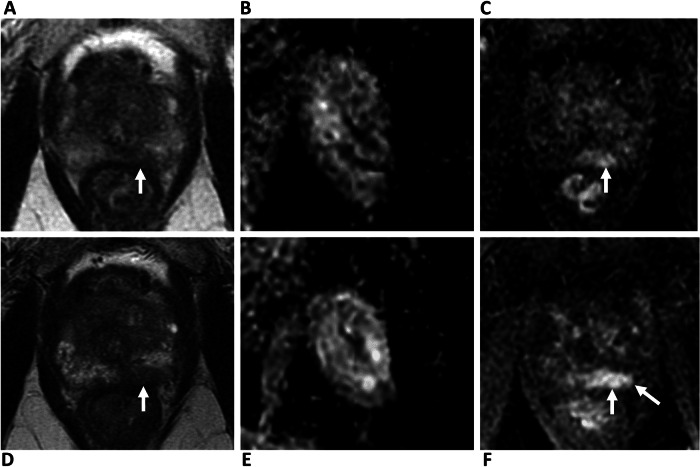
Value of DCE in patients with poor quality DWI. 71-year-old patient with a left THR, presenting PSA 6.01 ng/mL. A–C Baseline MRI: 14 × 5 mm PI-RADS 4 lesion in the medial left apex PZ with focal low T2 signal (A), nondiagnostic DWI due to THR (B), and focal early enhancement on DCE (C). Targeted biopsy shows Gleason score 3 + 4 = 7 (approximately 5% pattern 4), in 2/2 cores, 5 mm maximum tumour length. D–F MRI at 36 months with PSA 8.63 ng/mL. Increase in conspicuity on T2 (D), DWI remains nondiagnostic (E), but with a clear increase in the degree of enhancement on DCE to 21 × 7 mm (F, arrows). PRECISE score 4. Repeat biopsy shows Gleason score 3 + 4 = 7 (40% pattern 4), in 2/2 cores, 8 mm max tumour length. The patient treated with external beam radiotherapy
